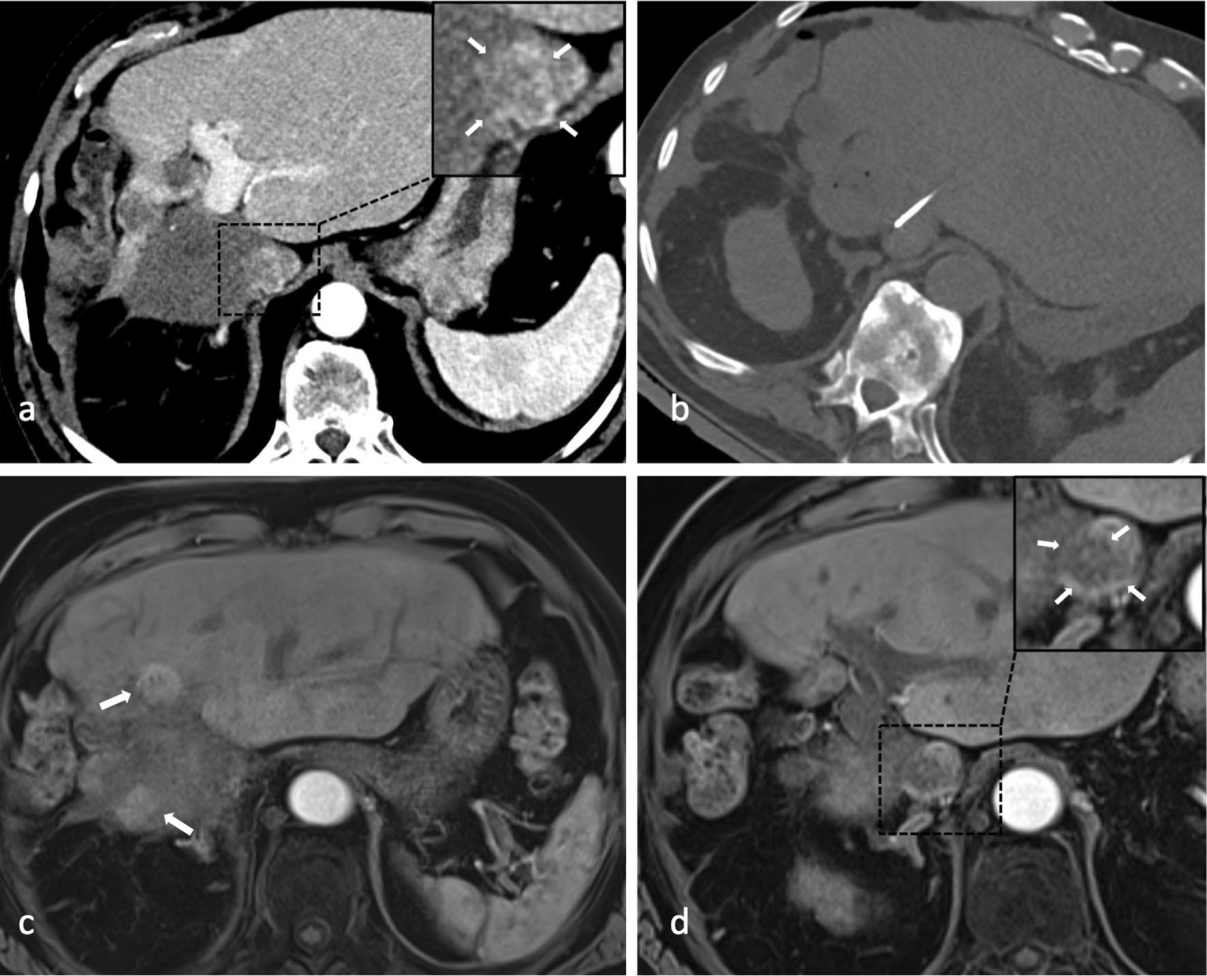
A Arterial phase CT showing contrast enhancing tumor thrombus (magnified image in the right upper corner with white arrows) in January 2018. B Axial non-enhanced control CT depicting needle placement for ablation of residual tumor thrombus in inferior caval vein (March 2018); last intervention up until now. C, D MRI scan of last follow-up in June 2019 with two recurrent nodules adjacent to necrosis zone (white arrows in c) and partially devascularized residual tumor thrombus in inferior caval vein (magnified image in the right upper corner with white arrows in D)
